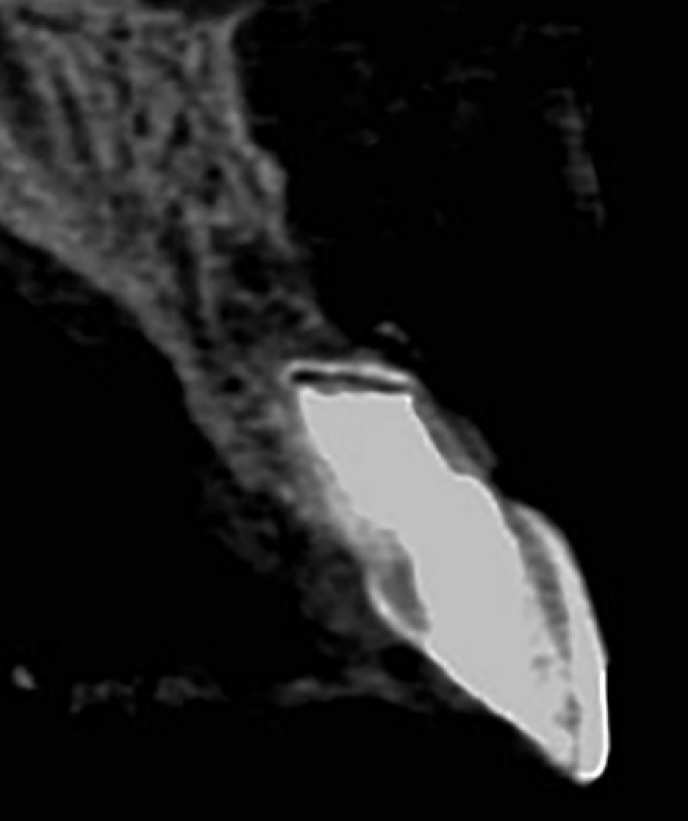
Cone-beam computed tomography (CBCT) image shows the complete healing of periapical lesion and PDL reformation 18 months after root-end filling with CEM cement
